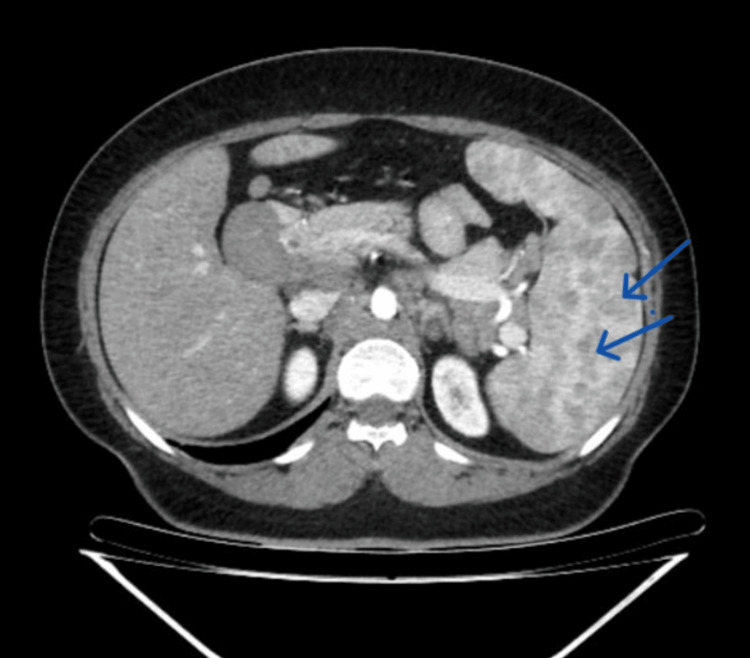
Abdominal CT scan. Abdominal CT imaging revealing splenomegaly with nodular confluent lesions (blue arrows).
